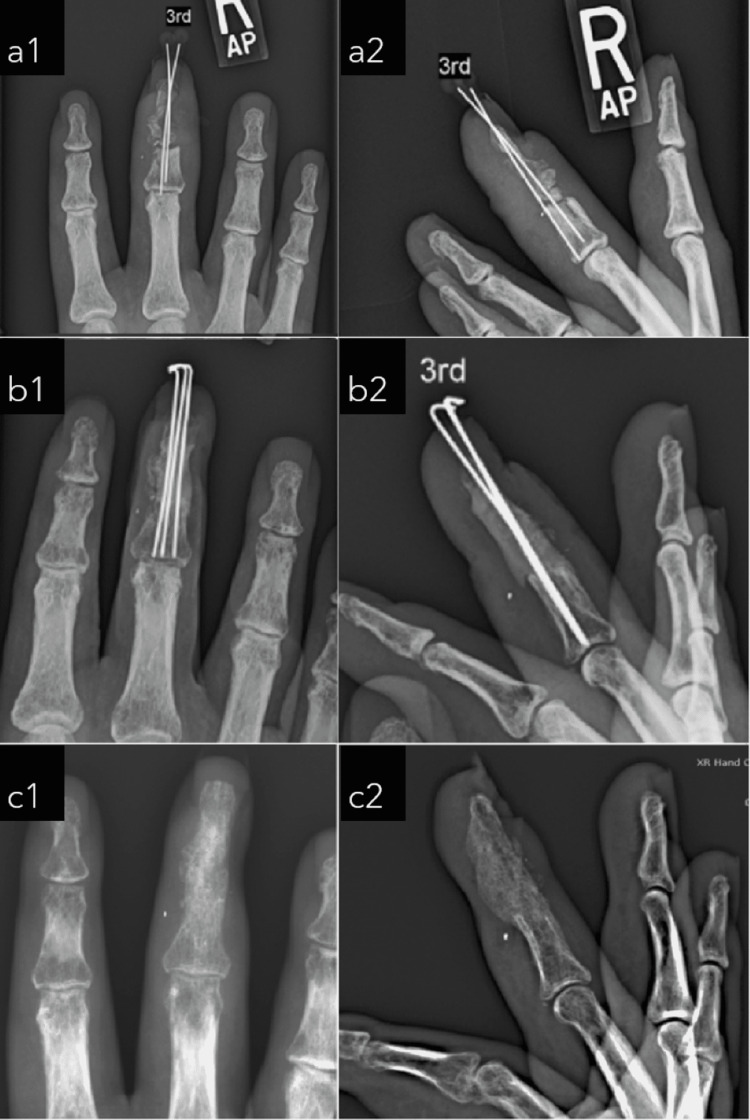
Posterior-anterior and lateral radiographs at preoperative (a1, a2), post first stage (b1, b2), and post second stage (c1, c2).
